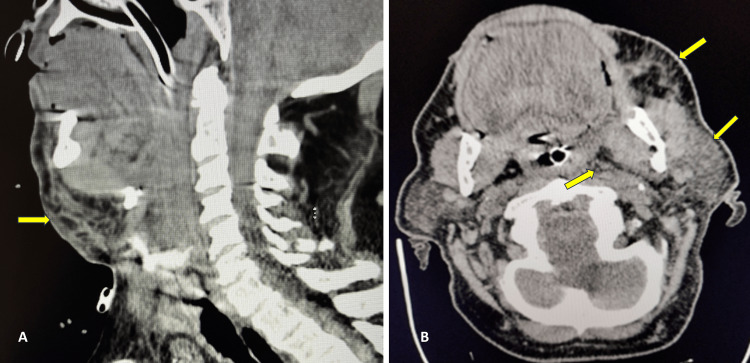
Soft tissue neck CT scans in sagittal (A) and axial (B) views, without contrast, revealing extensive soft tissue swelling extending from the left side of the face and the left submandibular region into the anterior neck There is notable swelling in the parapharyngeal space resulting in effacement of the airway. CT, computed tomography
